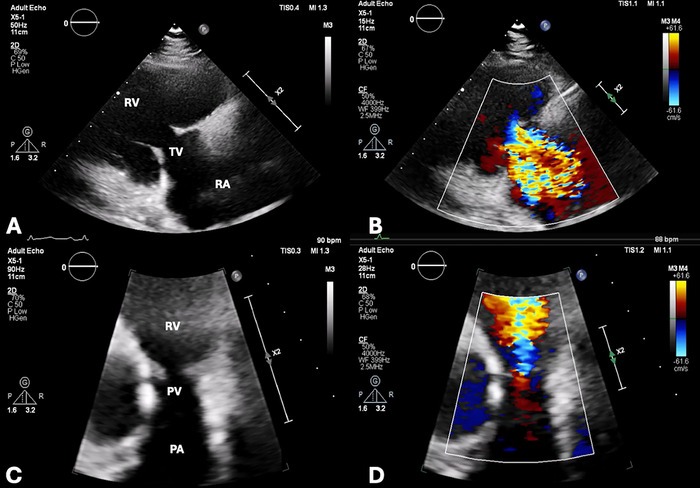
Transthoracic echocardiogram shows (A) thickened and restricted motion of tricuspid valve (TV) leaflets in the parasternal long-axis right ventricle (RV) inflow view, (B) severe tricuspid regurgitation demonstrated by color Doppler in the parasternal long-axis RV inflow view, (C) thickened and restricted motion of the pulmonic valve (PV) cusps in the parasternal short-axis view, and (D) severe pulmonic regurgitation demonstrated by color Doppler in the parasternal short-axis view. PA, pulmonary artery; RA, right atrium.
